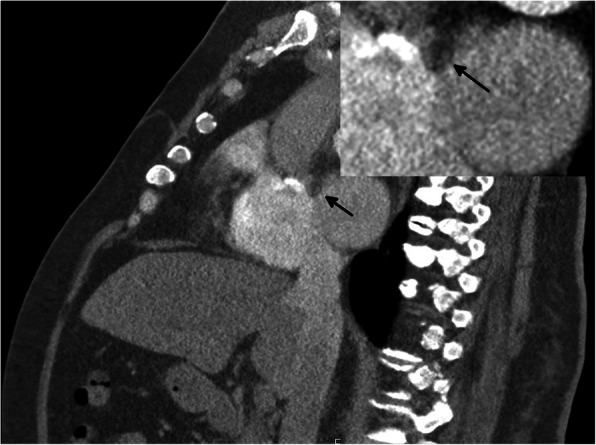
Chest computed tomographic image demonstrating a filling defect adjacent to the interatrial septum (arrow)
